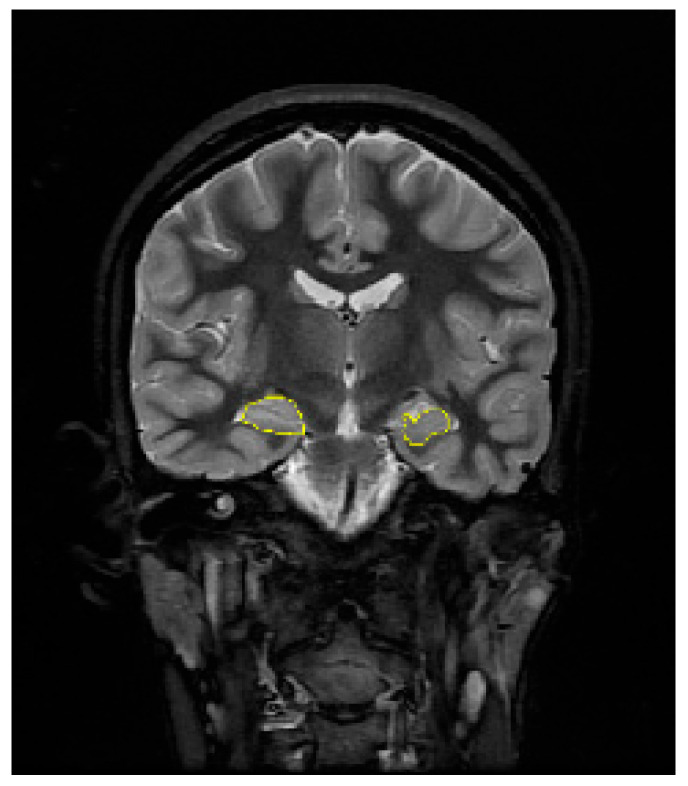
MRI slice of a TLE patient with hippocampus boundaries drawn in yellow by an expert.
